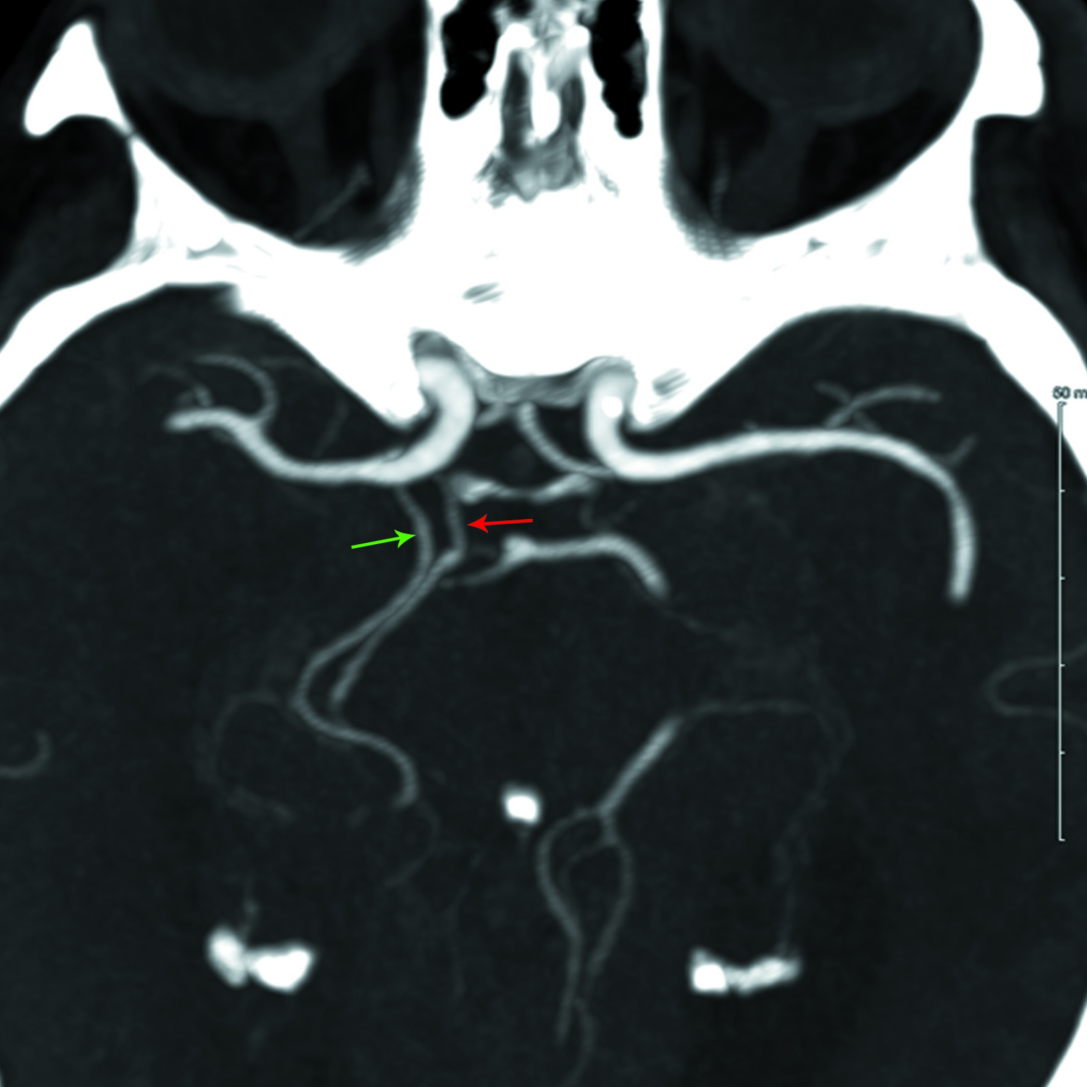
Axial MIP reformatted images from CT angiogram of the head showing two vessels arising from the posterior aspect of the distal ICA The proximal (more medially-oriented) vessel (red arrow) courses posterior, paralleling the fetal right PCA (green arrow) until the P2/3 segment. MIP = maximal intensity projection; CT = computed tomography; ICA = internal carotid artery; PCA = posterior cerebral artery
